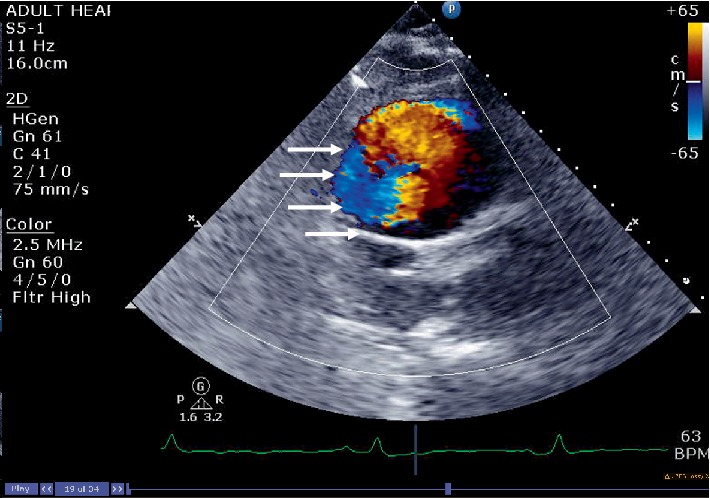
Color Doppler helps in appreciating the true lumen (arrows) from the false lumen.
